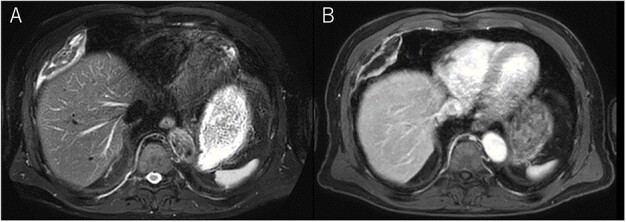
Chest MRI; (A) T2-weighted imaging showed several high signal foci in the tumor; (B) irregular marginal enhancement was observed.
